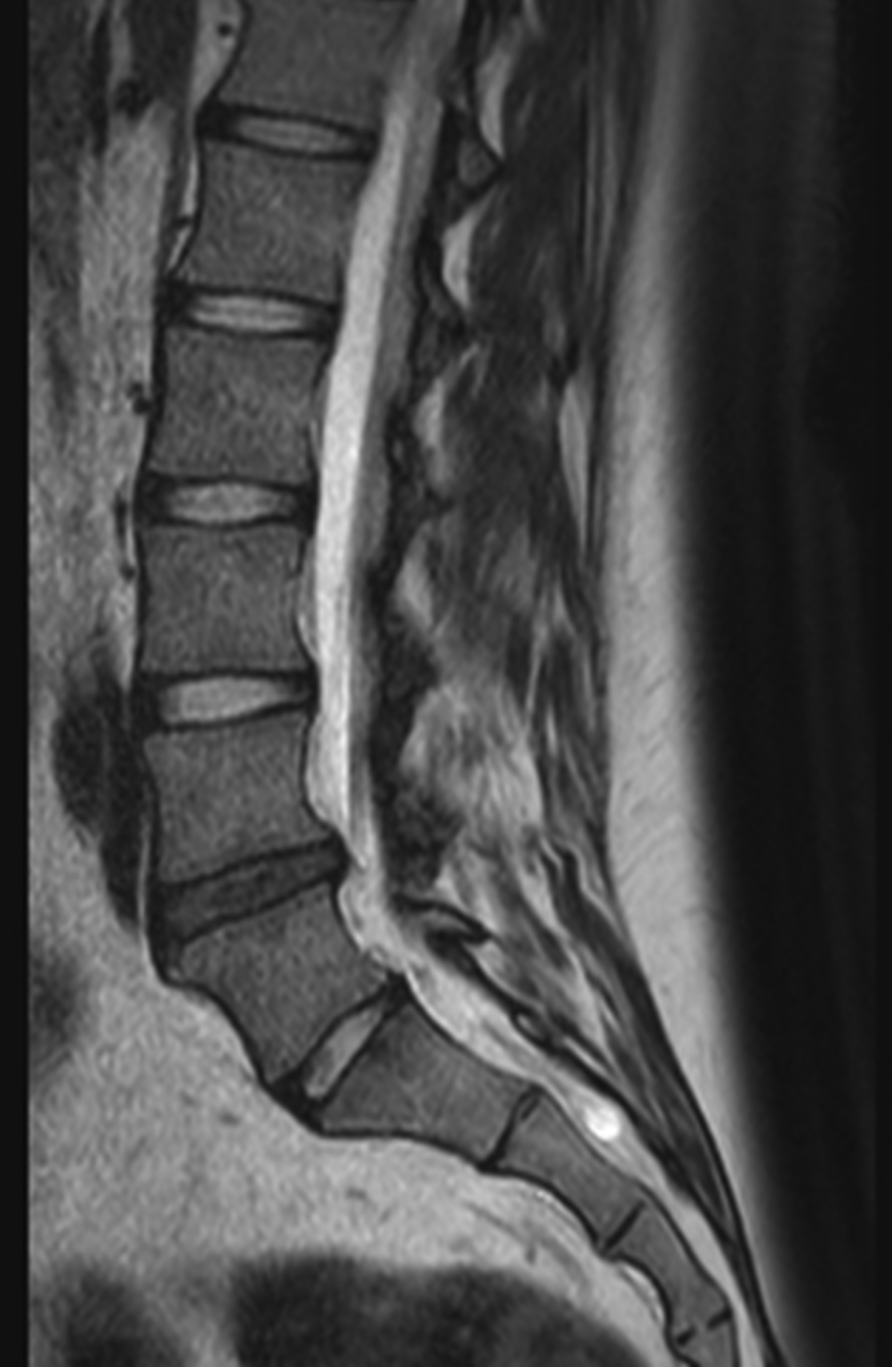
T2‐weighted magnetic resonance imaging (MRI) sagittal reconstruction of a 22‐year‐old female with persistent LBP for 8 months despite conservative treatment and IVD degeneration at the L4/5 level Pfirrmann grade III without endplate changes
